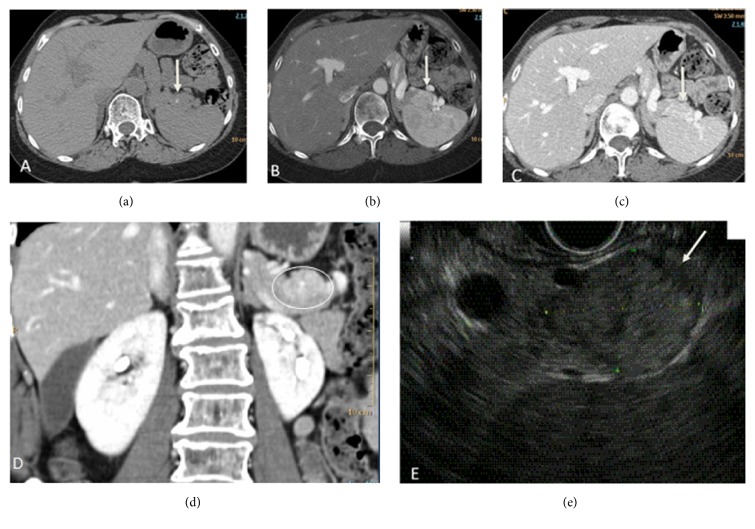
Axial noncontrast CT (a) shows a small calcification at the tail of the pancreas. Axial arterial phase CT (b) shows a 2.5 cm hyperenhancing pancreatic mass which on venous phase ((c) and (d)) shows slightly more heterogeneity compared to the spleen. Endoscopic ultrasound (e) showed heterogeneous hypoechoic 1.7 x 2.5 cm pancreatic tail mass with calcifications.
